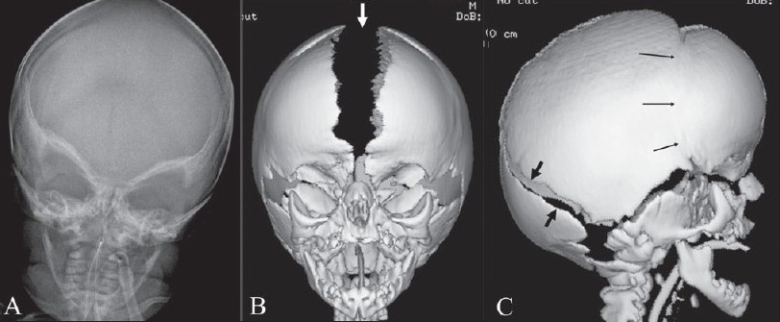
Harlequin appearance. Frontal view of the skull (A) in a child with Apert syndrome shows elevated superolateral angles of both orbits giving the appearance of a ‘harlequin mask.’ Frontal (B) and lateral (C) surface-shaded display 3D CT views of the skull show the harlequin appearance of the orbits. The sagittal suture (arrow in B) and the lambdoid suture (black arrows in C) are wide open. The coronal suture (thin arrows in C) is fused, which is suggestive of coronal craniosynostosis
